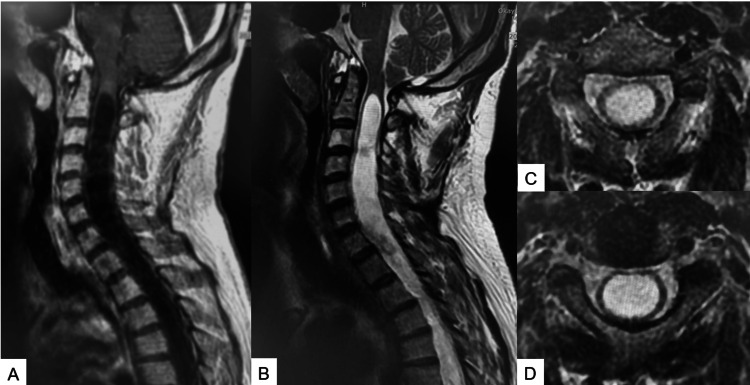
Preoperative MRI: (A) T1 sagittal MRI, (B) T2 sagittal MRI, (C) T2 axial MRI at C4, and (D) T2 axial MRI at C5/6. MRI: magnetic resonance imaging
